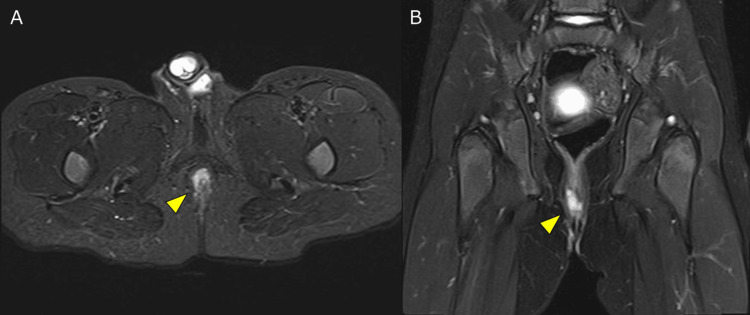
Results of MRI Short T1 inversion recovery MRI of the buttocks showing complex anal fistulas and a perianal abscess (yellow arrowhead): (A) Sagittal view. (B) Coronal view.
